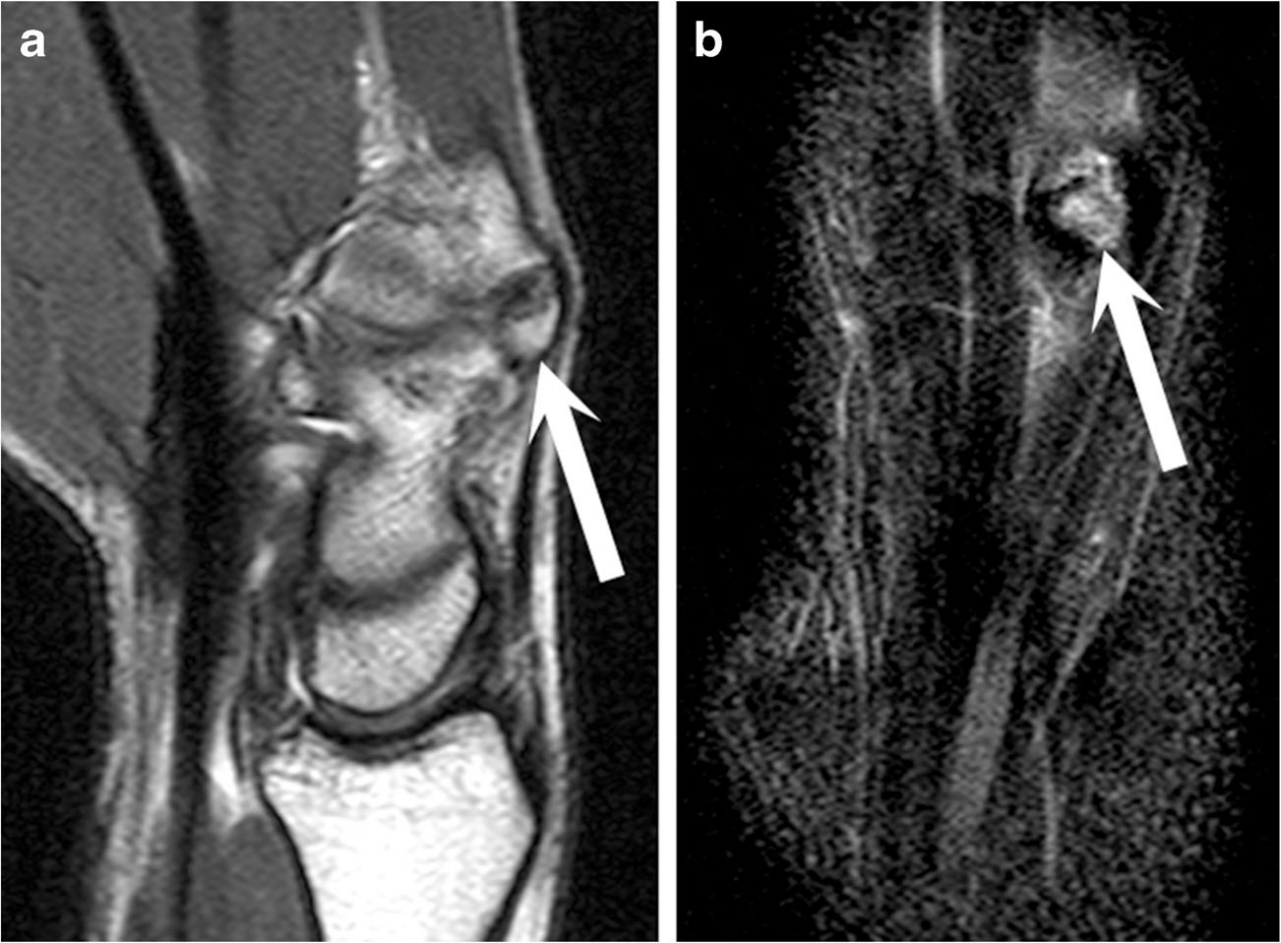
A 23-year-old professional male ice hockey player with persistent dorsal wrist pain. a T1-weighted and b T2-weighted fat-saturated sagittal MR images demonstrate an unfused carpal boss, i.e., os styloideum with marked bone marrow edema (*arrow*)
